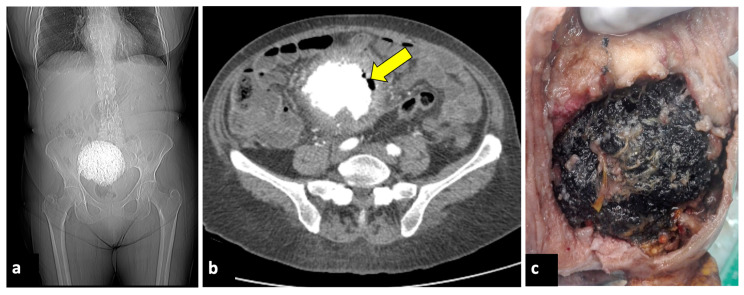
Pyomyoma in an eighty-year-old woman with a history of diabetes and steroid treatments who presented at the emergency department with fever, leucocytosis, and abdominal cramps for a week. A previous X-ray (a) shows a calcific uterine myoma. Axial CT scan (b) demonstrated the presence of air within the myoma (yellow arrow), eliciting the suspicion of an infected myoma. The patient underwent a hysterectomy (c), and the pathological findings confirmed the diagnosis of pyomyoma.
